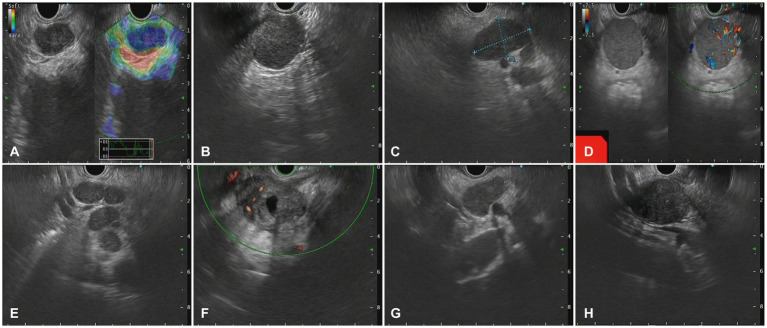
The Endoscopic ultrasonography image features in patients with metastatic tumors. (A) A case of cholangiocarcinoma. EUS showed multiple hypoechoic mass shadows, Doppler showed no blood flow signal, and the texture of elastic imaging was hard. (B) A case of gallbladder carcinoma. EUS showed low echo fusion lymph node shadow (C) A case of lung cancer. EUS showed multiple uniform hypoechoic nodules, some of which were fused with unclear boundaries, Doppler imaging showed no obvious blood flow signal, and the texture of elastic imaging was hard. (D) A case of liver cancer. EUS showed a class of circular lesions with uniform hypoechoic changes and Doppler blood flow signals. (E) A case of colon cancer. EUS showed multiple hypoechoic nodules, some of which were fused into clusters, with clear boundaries and homogeneous internal echoes. (F) A case of gastric cancer. EUS showed multiple hypoechoic nodules, which were round, with clear boundaries, anechoic, and non-uniform echoic necrosis. (G) A case of thymic carcinoma. EUS showed multiple enlarged lymph nodes with clear boundaries, and no obvious blood flow signal was observed by Doppler. (H) A case of rectal cancer. EUS scan showed circular hypoechoic lesions with partial fusion, and the Doppler scan showed no obvious blood flow signal.
